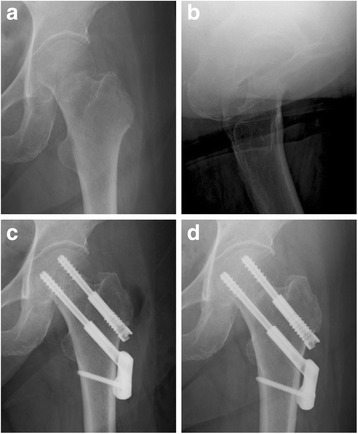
Plain radiographs demonstrating a femoral neck fracture fixed with Dual SC Screws. The screws were positioned adequately, and the bone union was obtained with short sliding length. (a, b preoperative, c postoperative, d 6 months postoperatively)
